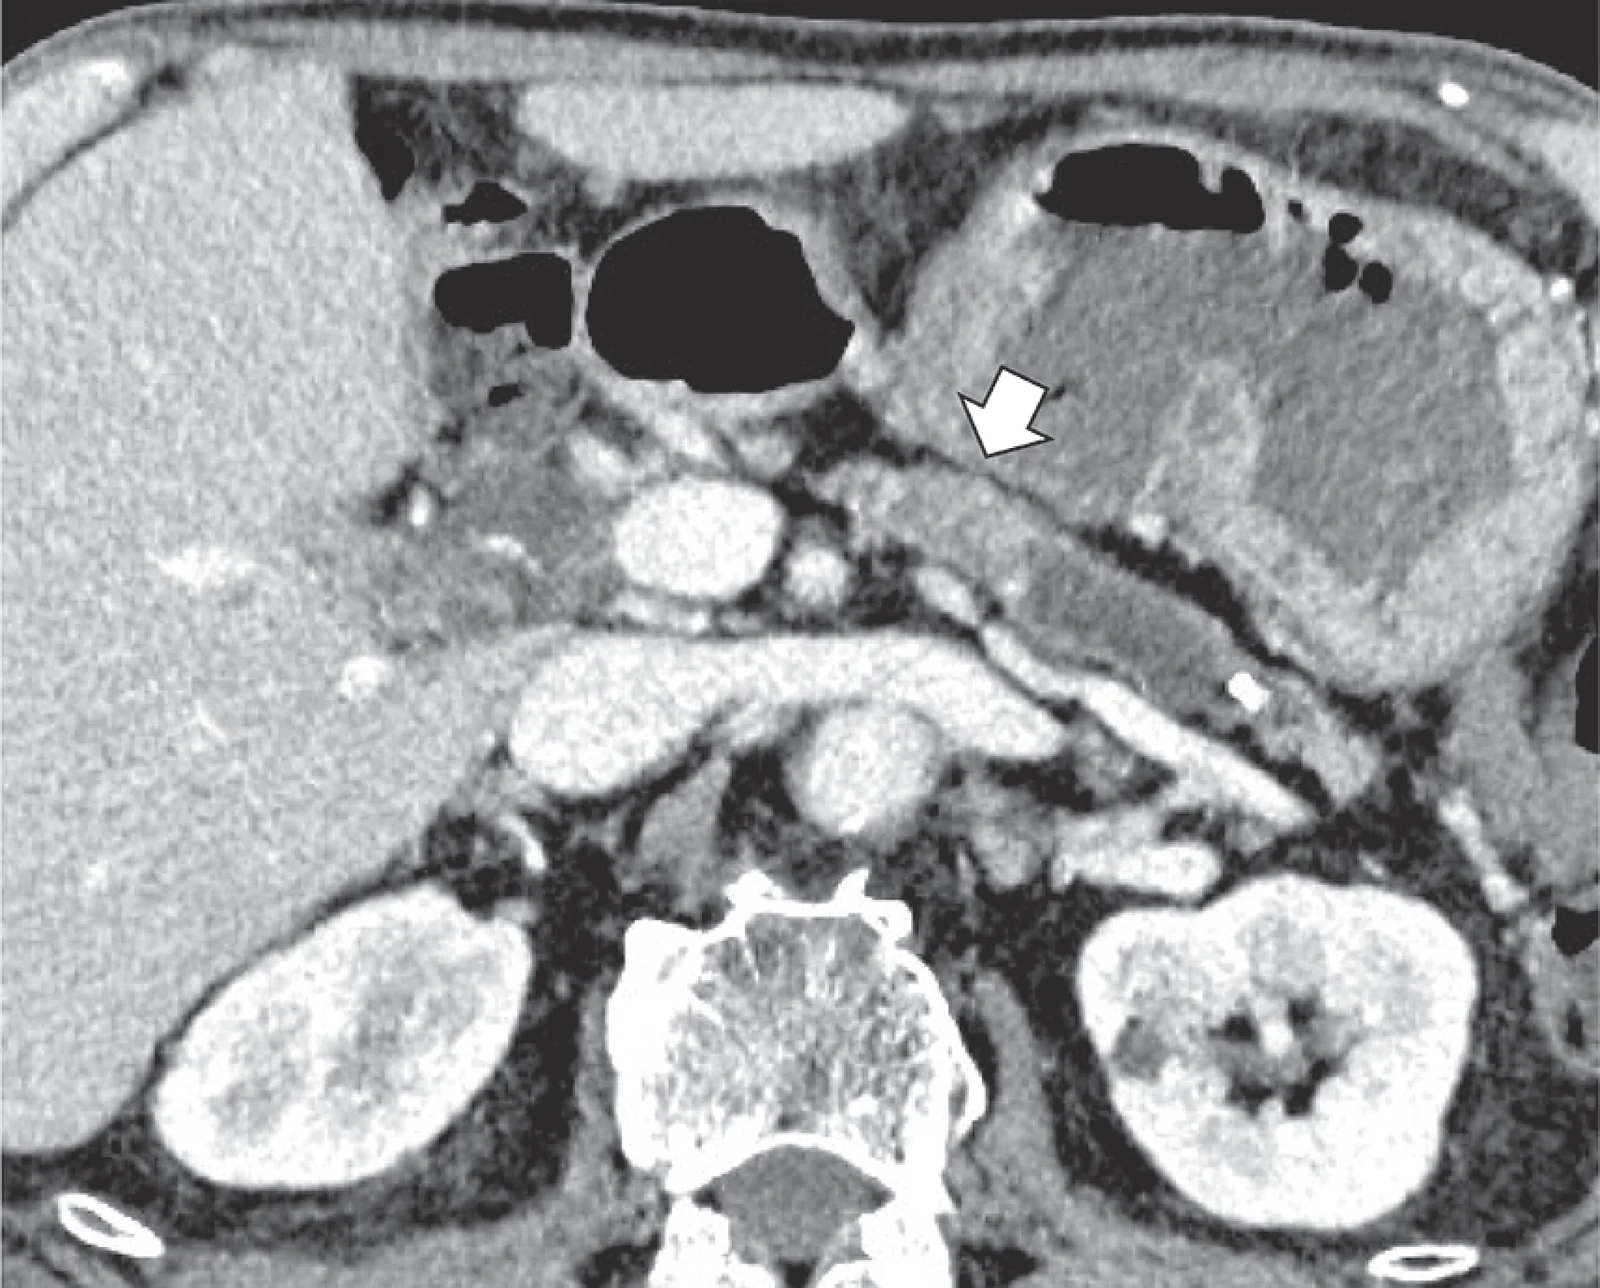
Contrast-enhanced computed tomography revealed a hypovascular
